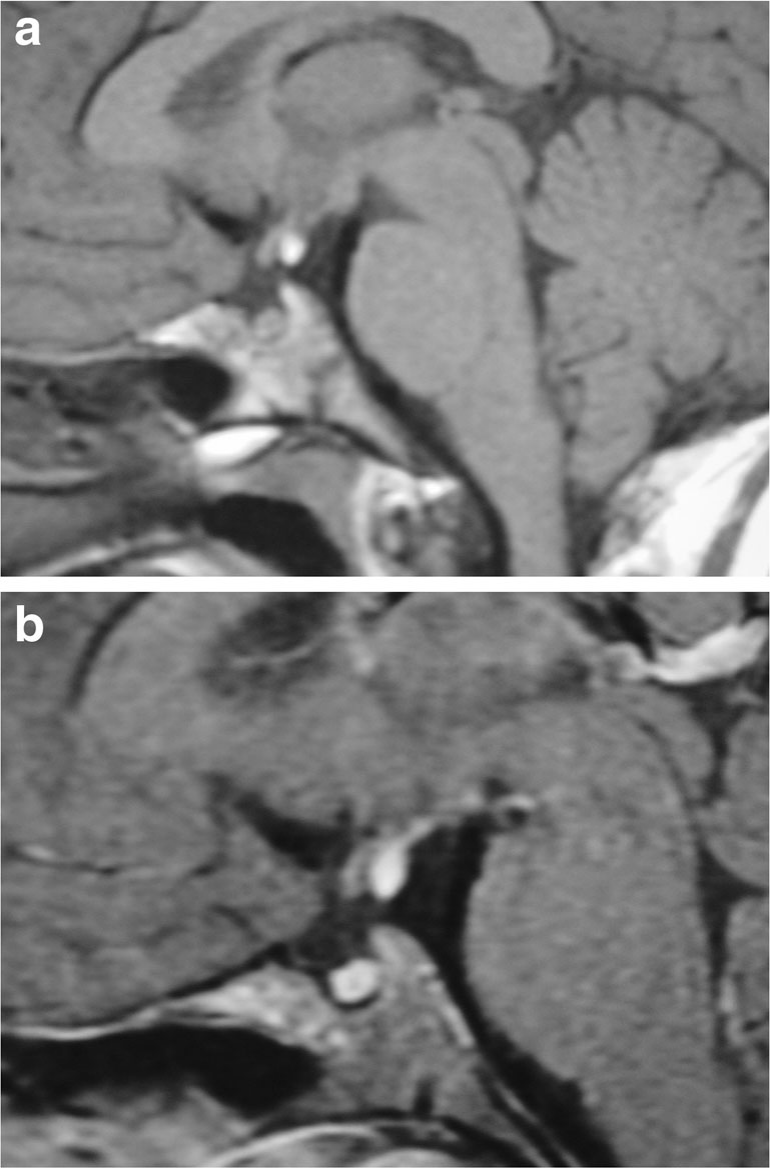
Ectopic posterior pituitary gland. Pre-contrast (a) and post-contrast (b) MR images show a normal anterior pituitary gland with contrast enhancement and an ectopic posterior pituitary located adjacent to the optic chiasm
